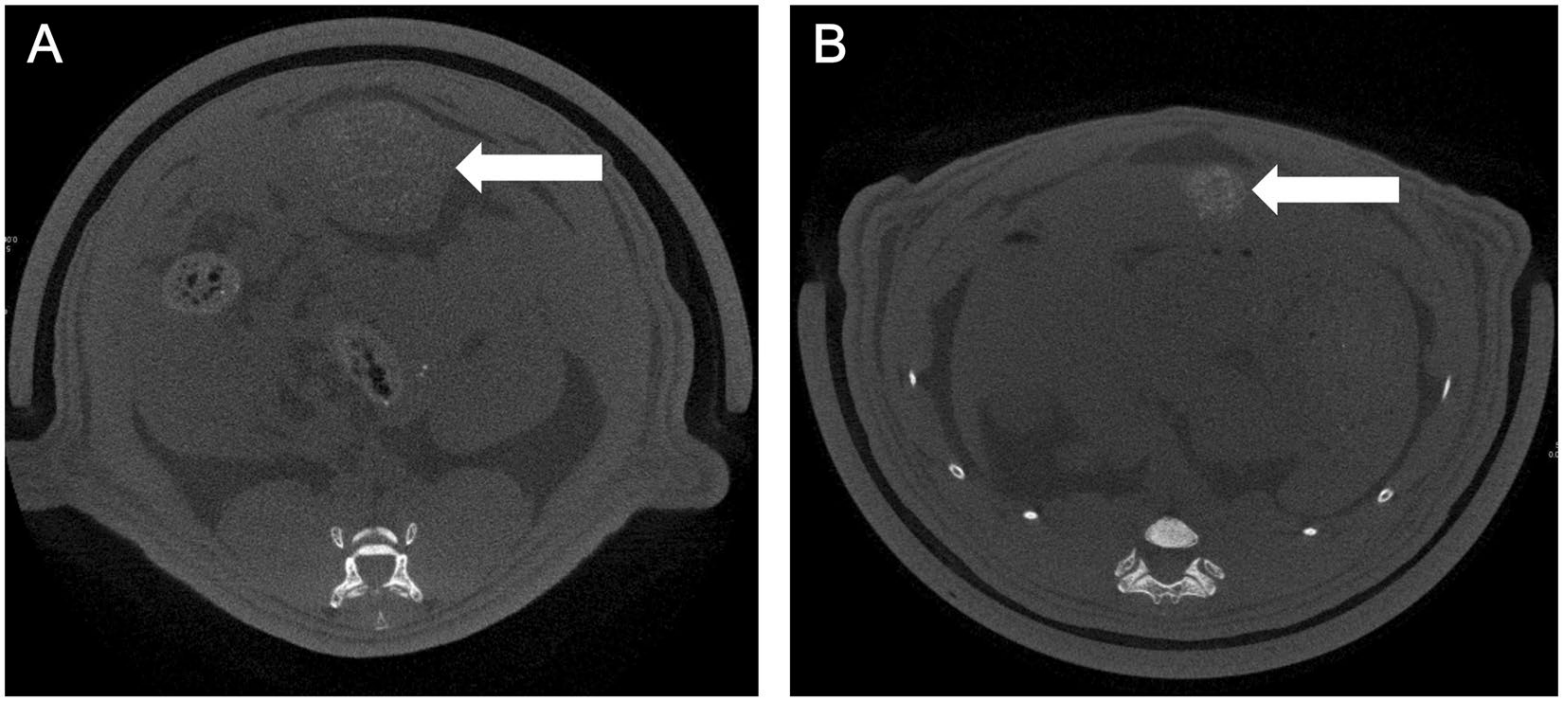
Hyper-attenuated spots appeared in the index tumor after IA administration of SOF/TIBA/PLGA20 MSs on CT images obtained on day 1 (A) and day 7 (B).
